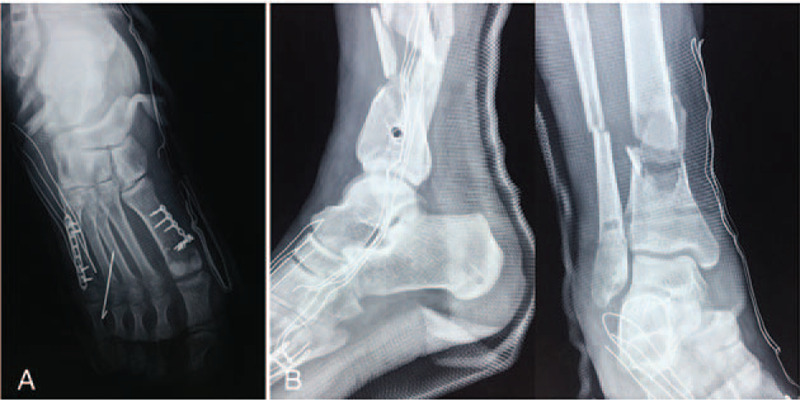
Digital radiograph of the patient's right foot after open reduction internal fixation (ORIF) and external fixator removal. ORIF was applied to the first and fifth metatarsals, and the fourth metatarsal was fixed by percutaneous K-wire insertion (A). The remaining 2 fractured metatarsals, tibia, and fibula were fixed by plaster instead of an external fixator (B).
